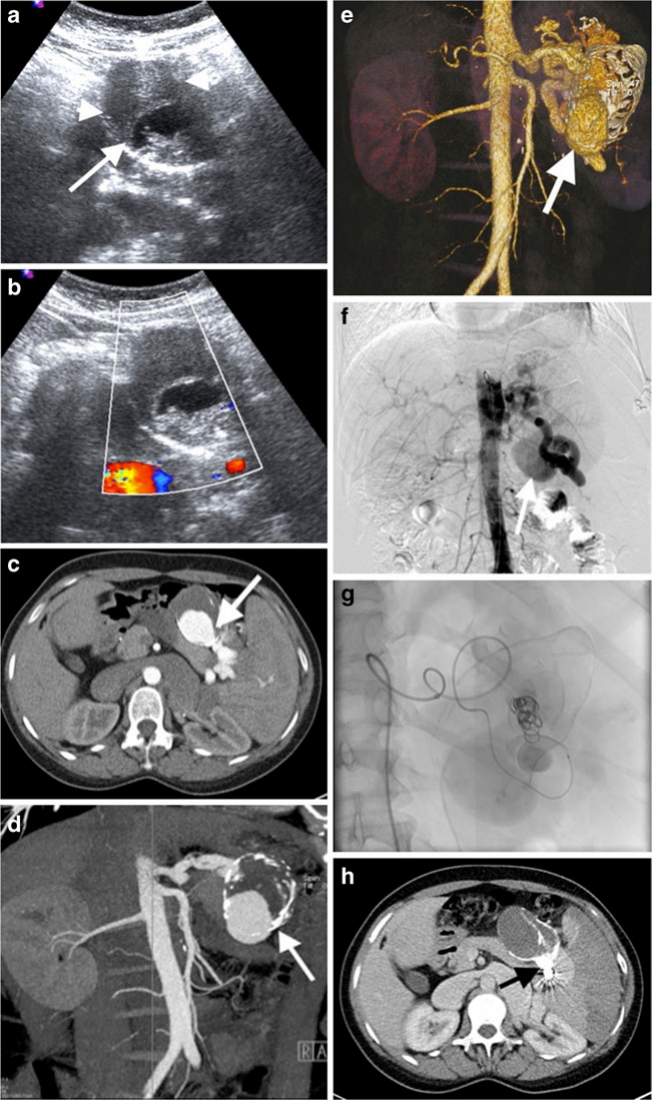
Splenic artery aneurysm. (a) Gray scale US of a 45-year-old woman presenting with left upper quadrant pain demonstrates a well-defined mass, with anechoic (*arrow*) and hypoechoic (*arrowheads*) portions representing patent and thrombosed parts of the aneurysm, respectively. (b) No flow was detected on colour flow Doppler US. (c) Axial contrast-enhanced and (d) coronal maximum intensity projection (*MIP*) CT demonstrates splenic artery aneurysm (*arrows*) with partial thrombosis. (e) Volume rendering (*VR*) CT image reveals splenic artery aneurysm (*arrow*). (f) Digital subtraction angiography (*DSA*) demonstrates aneurysm (*arrow*) arising from splenic artery. (g) Coil embolization was performed to embolize the neck of the aneurysm. (h) No contrast enhancement was observed (*arrow*) on contrast-enhanced CT after coil embolization
